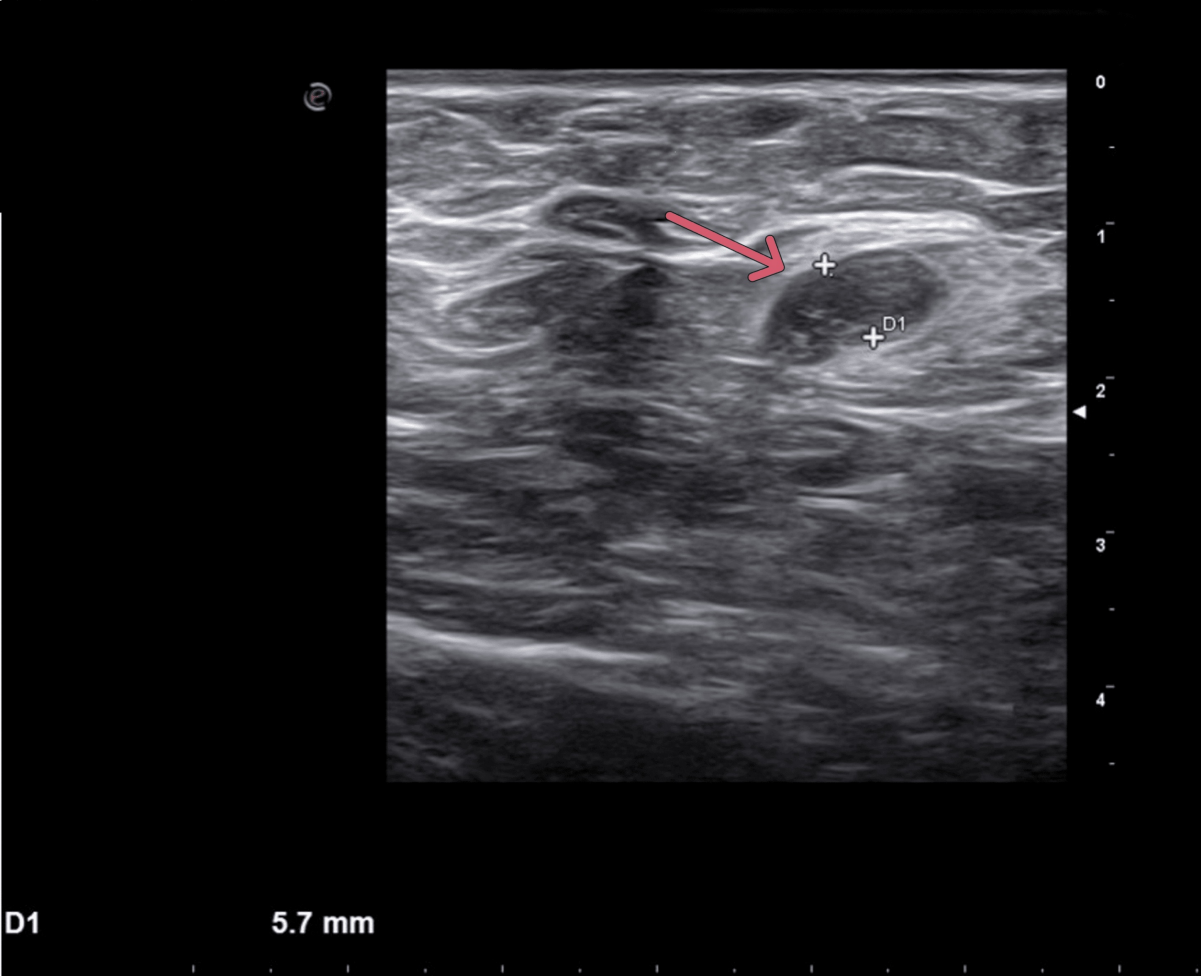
Axillary ultrasound Axillary ultrasound shows lymph nodes with suspicious features (the arrow indicates the area of interest).
